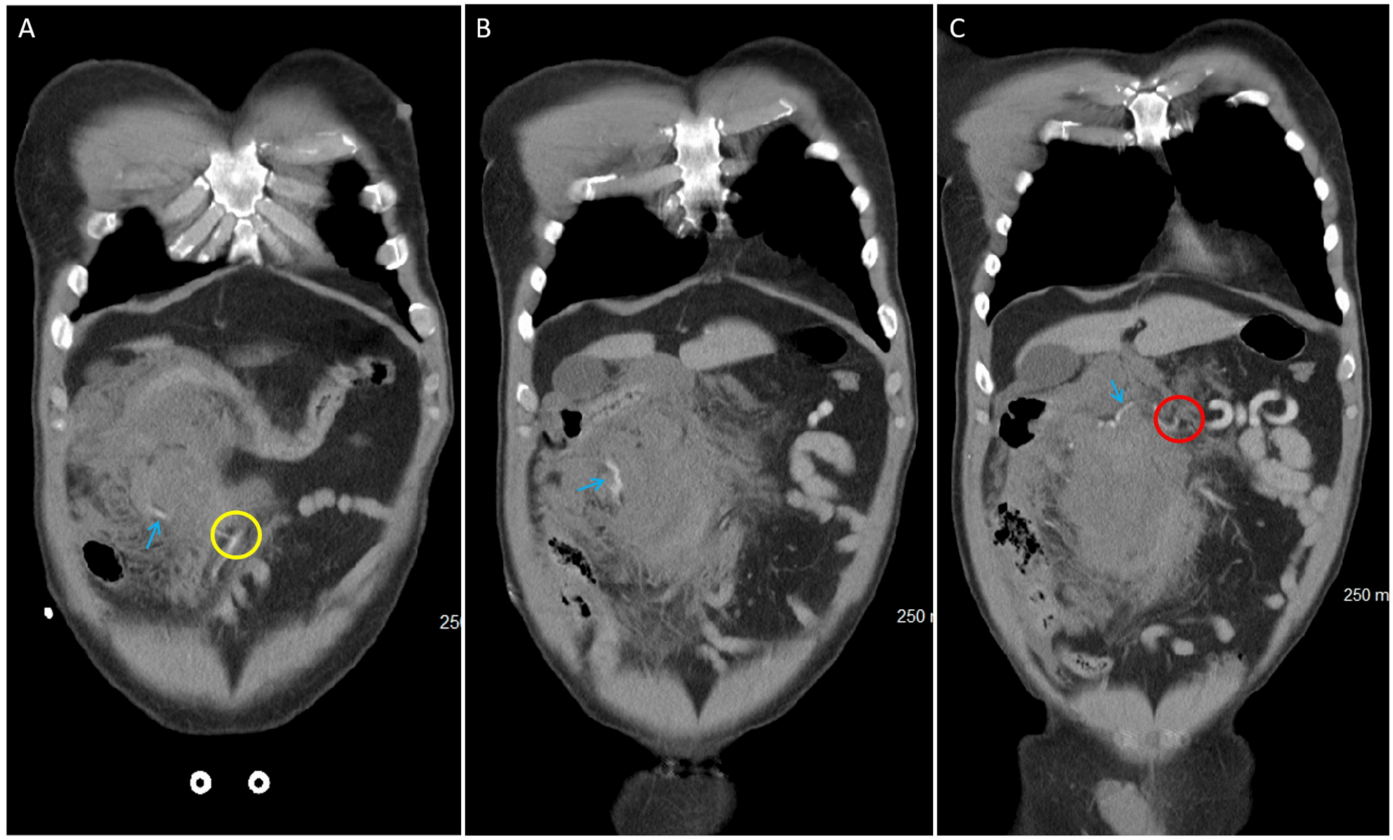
Coronal CT images from anterior to posterior, demonstrating the course of the arc of Buehler through the abdomen. (A) The anteriormost slice of the coronal imaging, showing the distal anastomosis to the SMA represented by the yellow circle with the arc of Buhler indicated by the blue arrow. (B) The mid-abdominal coronal imaging, demonstrating the course of the arc of Buhler as it progresses proximally indicated by the blue arrow. (C) The posteriormost slice from coronal CT imaging, demonstrating the proximal anastomosis with the celiac trunk indicated by the red circle with the path of the arc of Buhler indicated by the blue arrow Yellow circle: the arc of Buehler connection to SMA Red circle: the arc of Buehler connection to the celiac trunk Blue arrow: the arc of Buehler as it progresses through images CT: computed tomography; SMA: superior mesenteric artery
